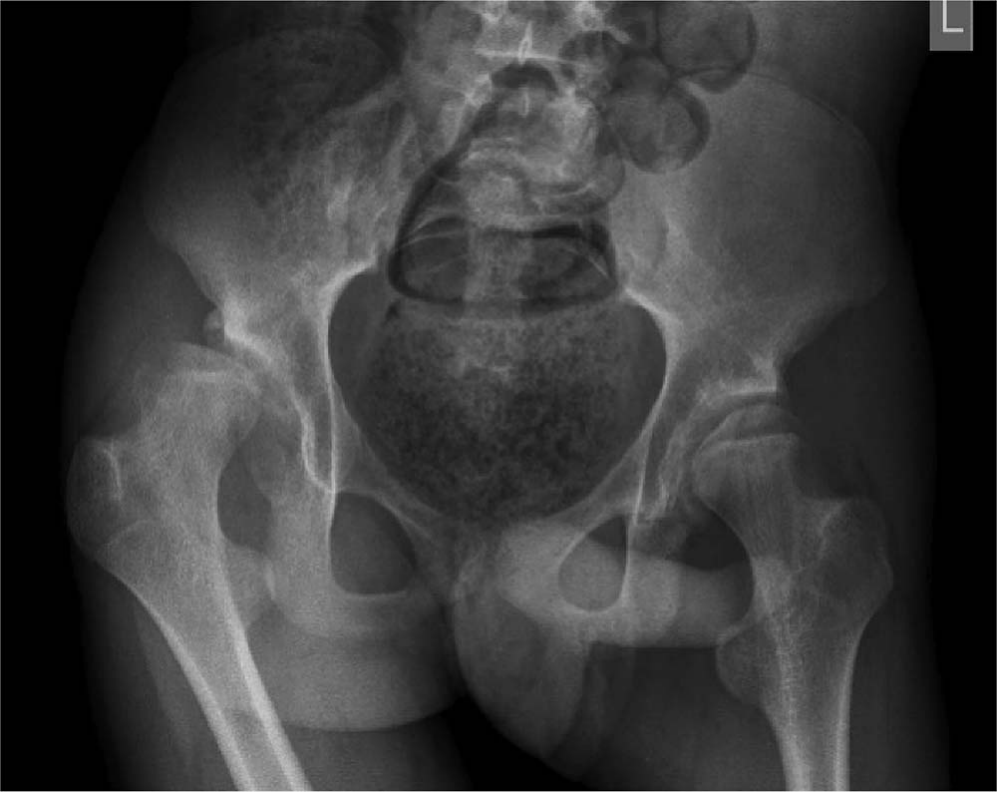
Case 2 pre-operative radiograph.
